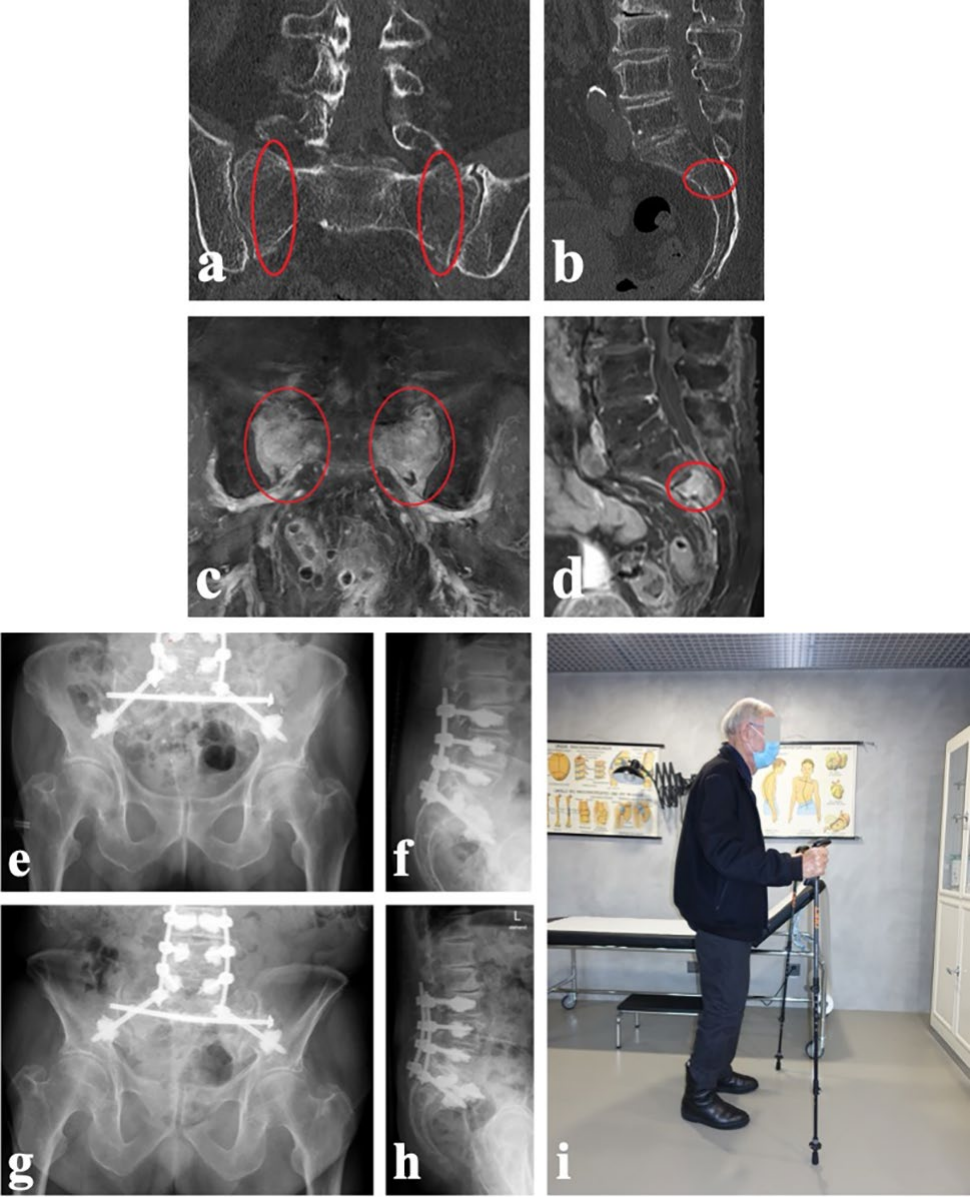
87-year-old male developed immobilizing low back pain due to multilevel lumbar degenerations and a non-displaced H-shaped sacral insufficiency fracture (FFP type IVb—spinopelvic dissociation). Coronal and sagittal reconstructions of computed tomography (a, b) and magnetic resonance imaging (c, d) (red circles show the fractures). Postoperative ap pelvis (e) and lateral radiograph of the lumbar spine (f) and the radiographs one year postoperatively (g, h) after cement-augmented percutaneous lumbopelvic and transsacral stabilizations with an excellent functional outcome (i)
